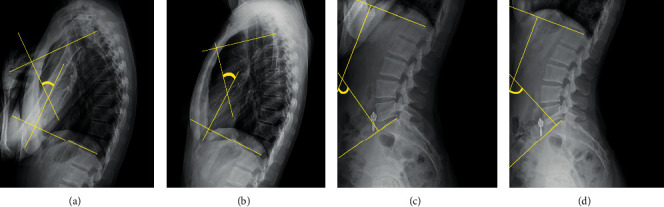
Lateral radiographs showing thoracic kyphosis (a), thoracic extension (b), lumbar lordosis (c), and lumbar extension (d). The line markers indicate the Cobb angle method used to take measurements in each position.
